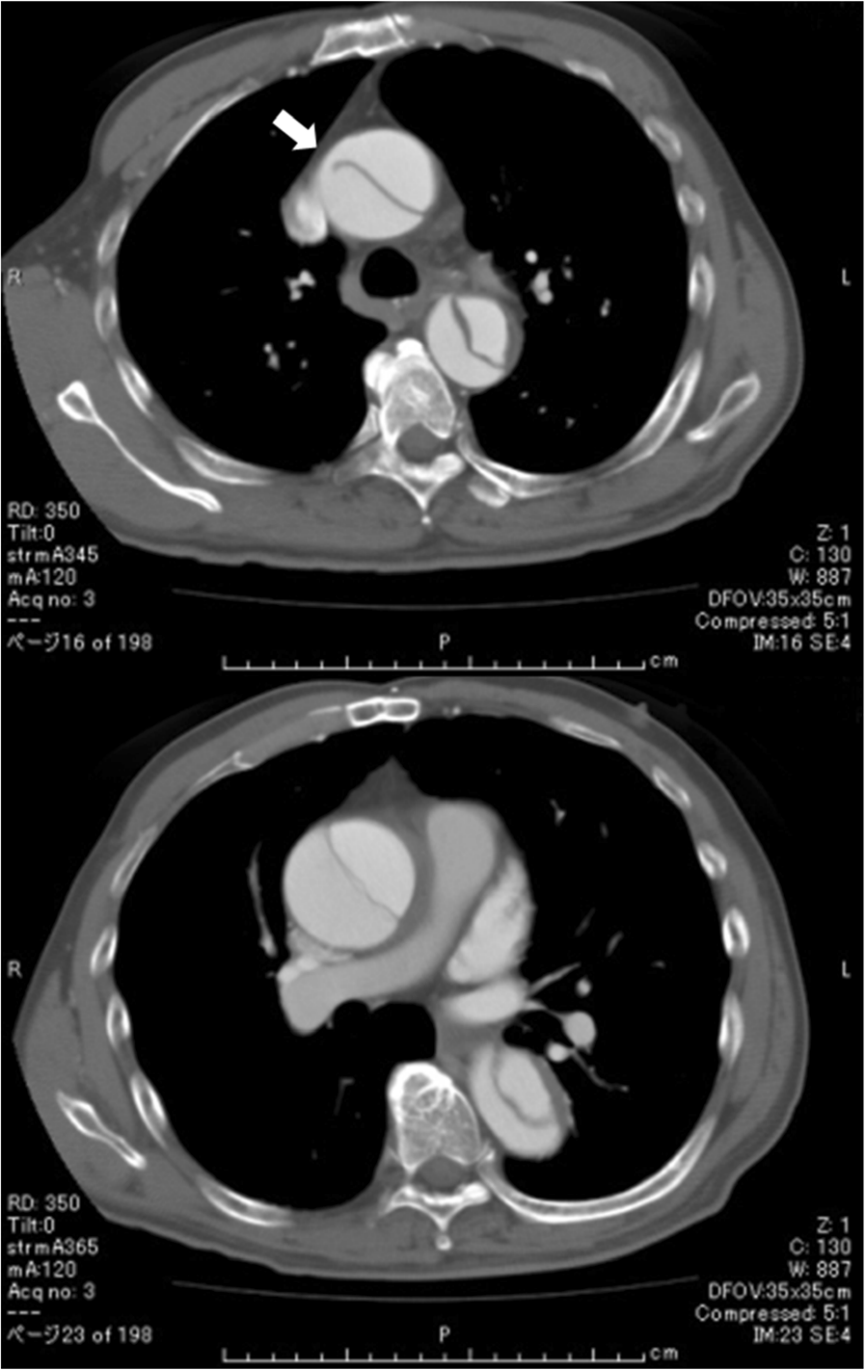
CTA showing intimal tear (arrow) and dissection of the ascending aorta.
